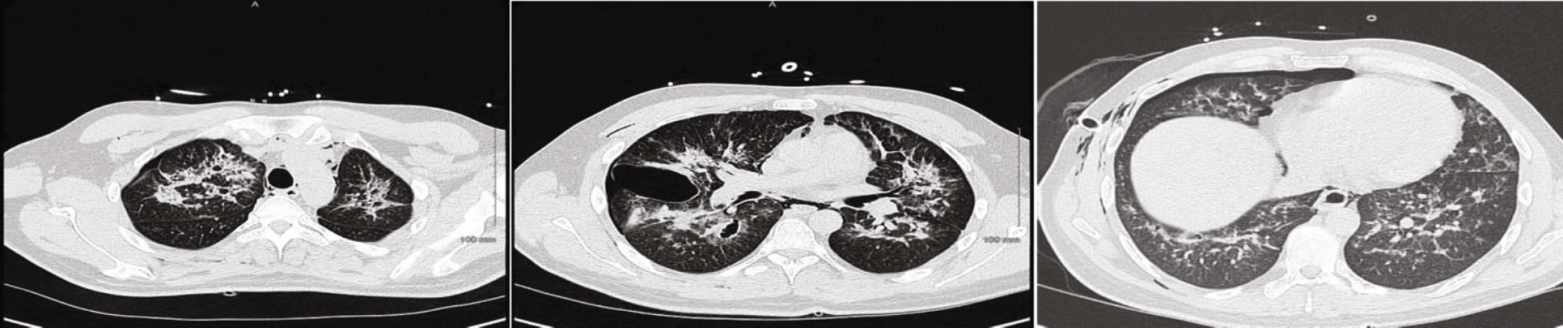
Chest CT showing a large bulla in the right lung adjacent to the major fissure with a few other bullae seen in the superior segment of the right lower lobe, pneumomediastinum, subcutaneous emphysema, and significant interstitial lung disease.
